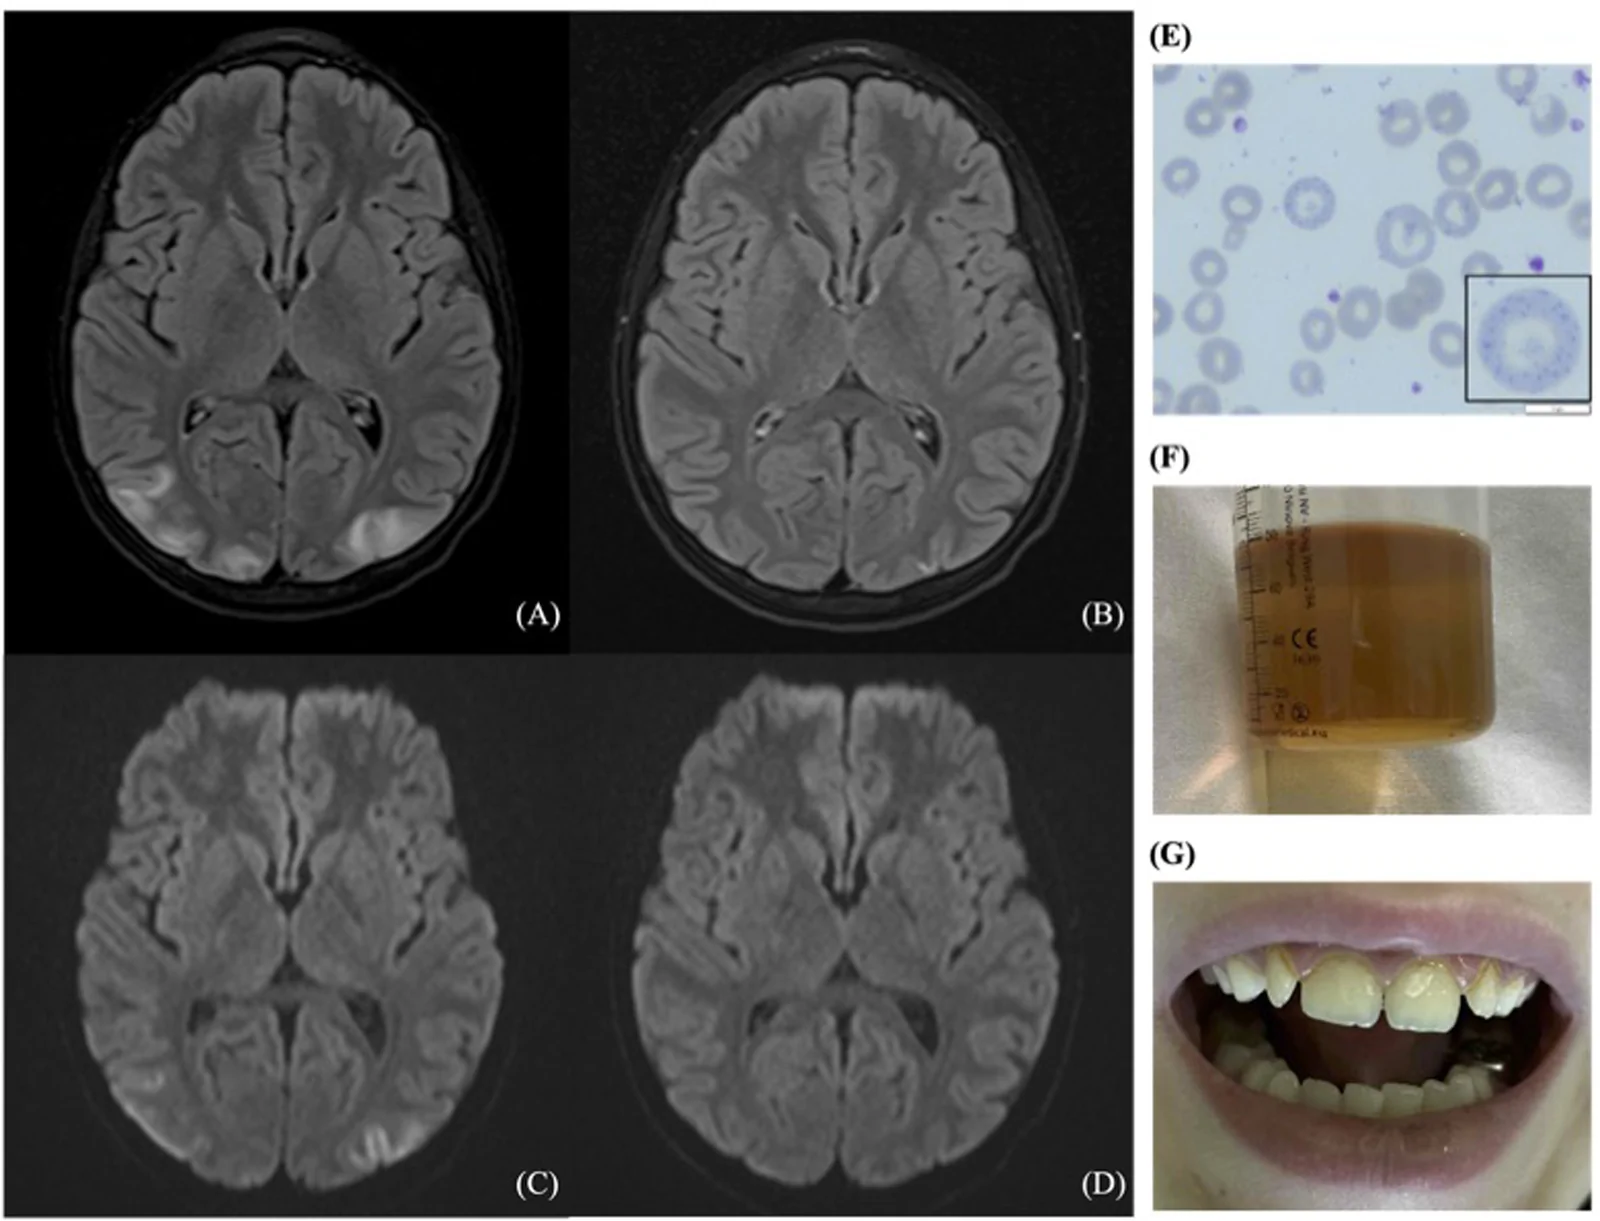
Pre- (A,C) and posttreatment (B,D) axial cMRI (1,5 T). *Top:* Fluid-attenuated inversion recovery (FLAIR) sequence. *Bottom:* diffusion weighted echo-planar imaging (EPI) sequence. Pretreatment images show cortical gray and subcortical white matter lesions in the occipital lobe with edema and sulcal effacement. Posttreatment images reveal near-complete resolution. Few focal cortical gray matter lesions in the left occipital lobe. (E) Blood smear showed basophilic stippling. (F) The patient's dark-colored urine after exposure to sunlight appears to be compatible with porphyria. (G) Discoloration of gingival margins.
